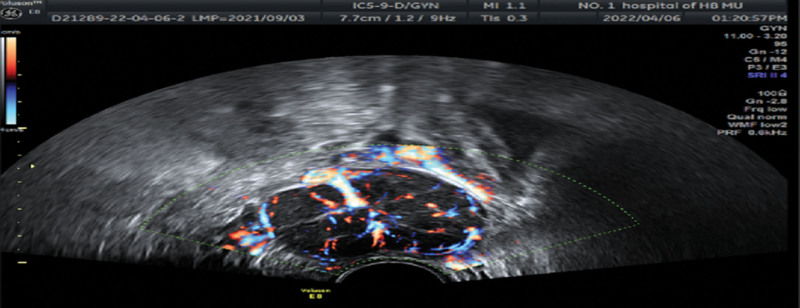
Transvaginal ultrasonography (case B) revealed a 4.6 × 3.5 × 3.3-cm solid mass with increased peripheral vascularity in the left ovary.
